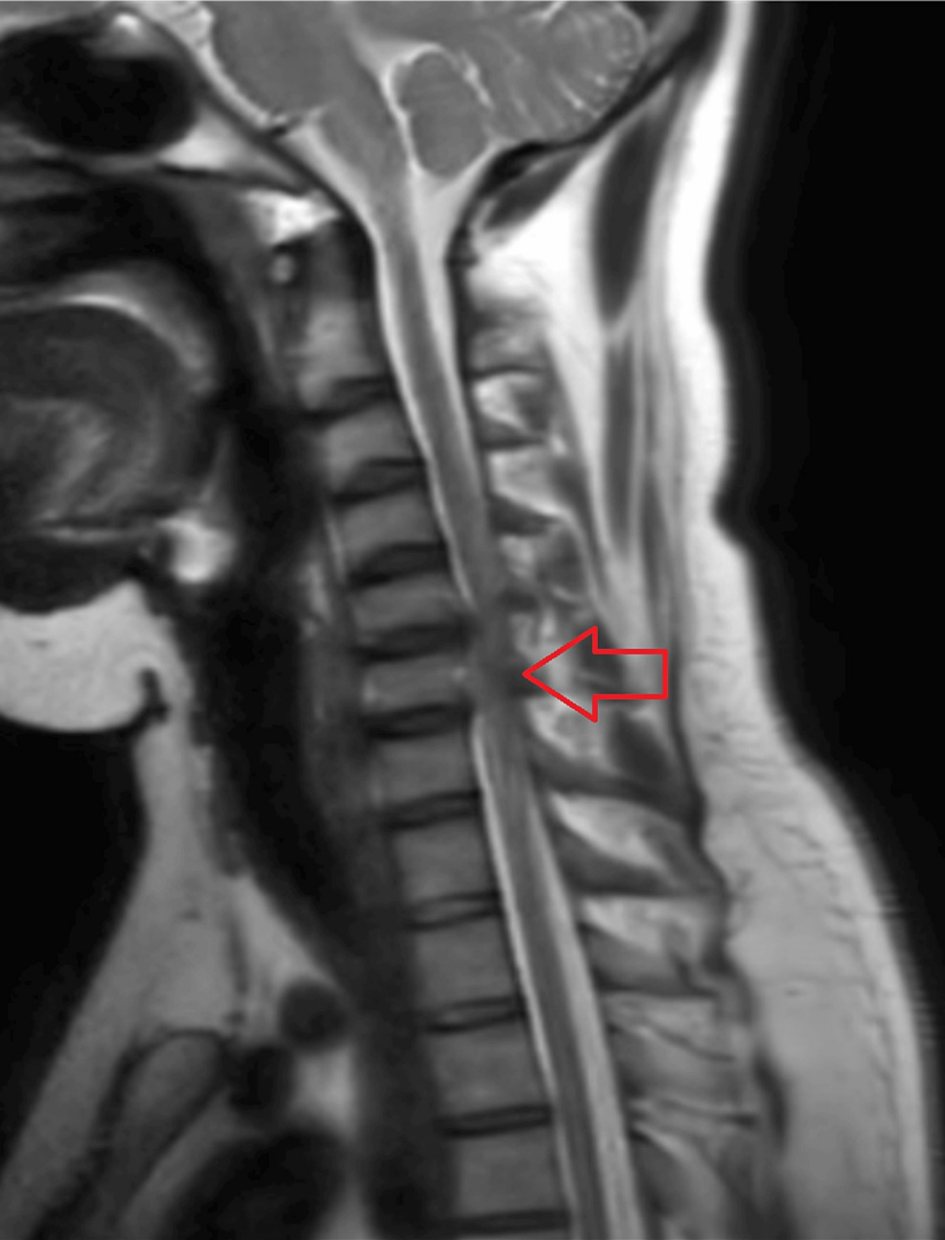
MRI of the cervical spine showing herniated discs at C4-C5 and C5-C6 causing stenosis (arrow) MRI: magnetic resonance imaging
